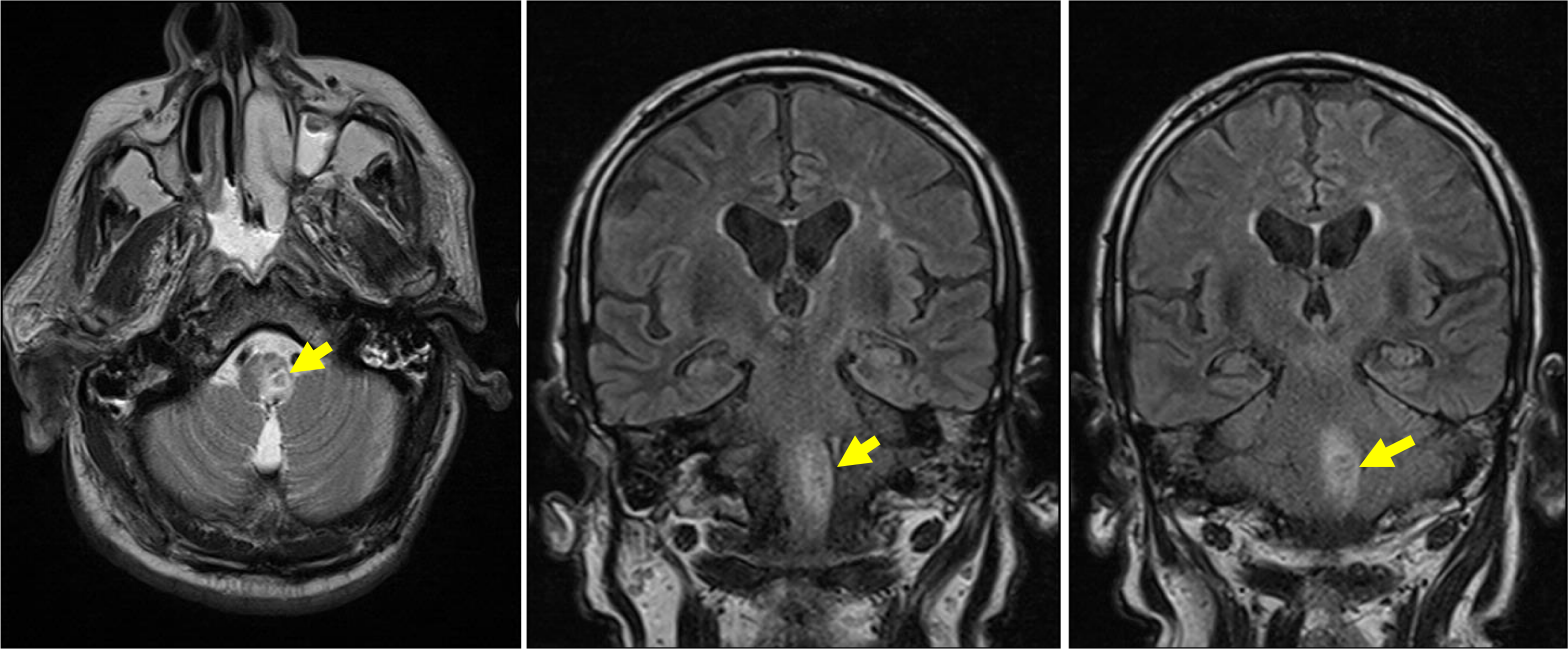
Subacute ischemic lesion in MRI T2 sequence.
